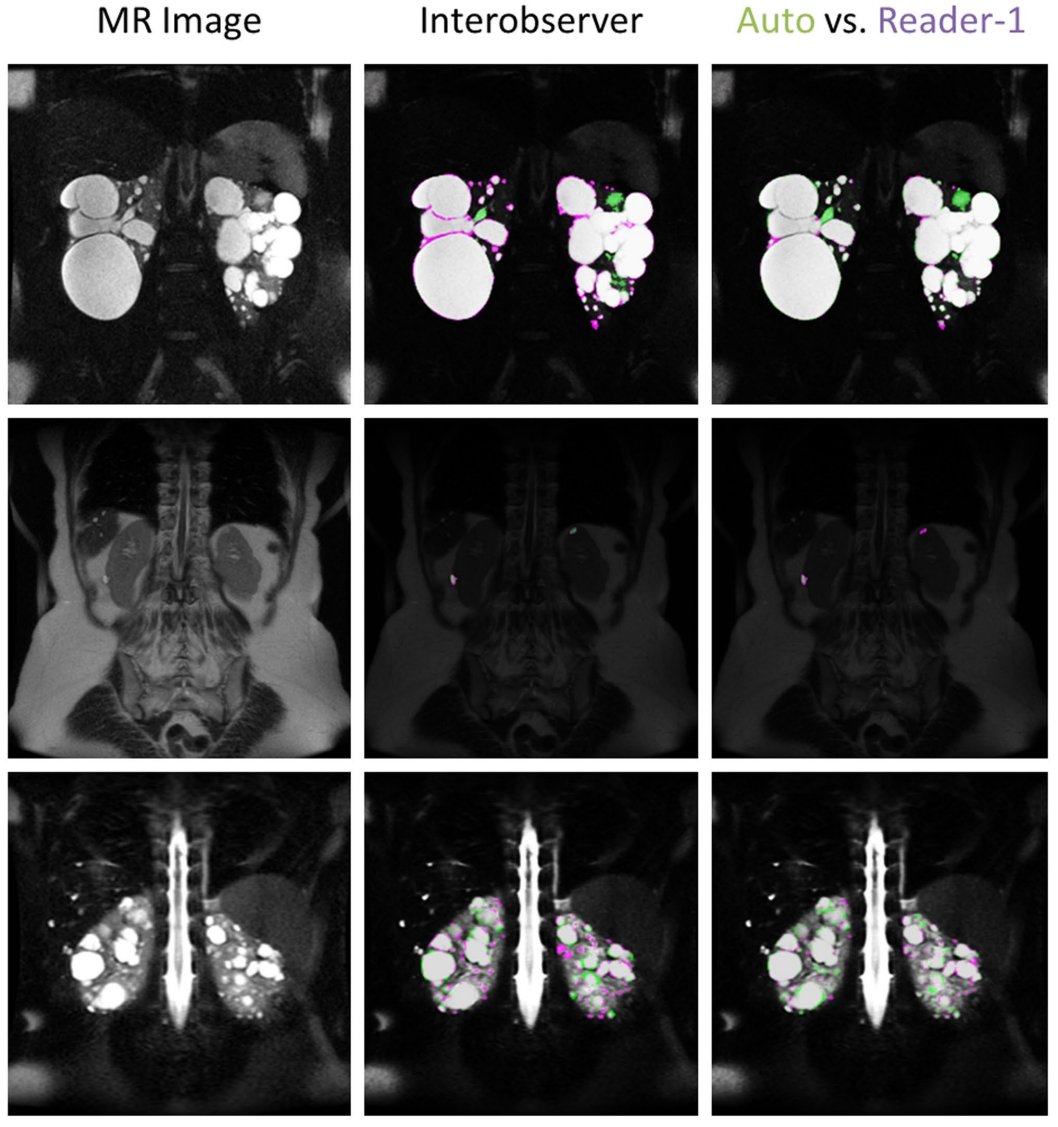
Visual comparisons between the interobserver segmentations and the automated approach compared to Reader-1. Shown in the left column are the MR images, the middle column are the gold-standard tracings comparing Reader-1 (violet) to Reader-2 (green), and right column compares Reader-1 (violet) to the automated approach (green). The top row highlights one of the best cases, with a Dice of 0.96 for interobserver, and 0.97 for the automated approach compared with Reader-1. The middle row is the worst case in terms of the automated methods performance, with an interobserver Dice metric of 0.66 and an automated Dice of 0.50 vs. Reader-1. The bottom row highlights a fairly typical case in terms of performance, with interobserver Dice of 0.84, and automated Dice of 0.86 vs. Reader-1. Regions that are seen to cause the greatest variability for both manual tracings as well as the automated approach are bright vessels, the renal pelvis, as well as complex cysts (appearing dark on the T2-weighted images). Agreement between the two is shown as dark gray/transparent. The background image is darkened in order to better visualize the segmentation overlap
